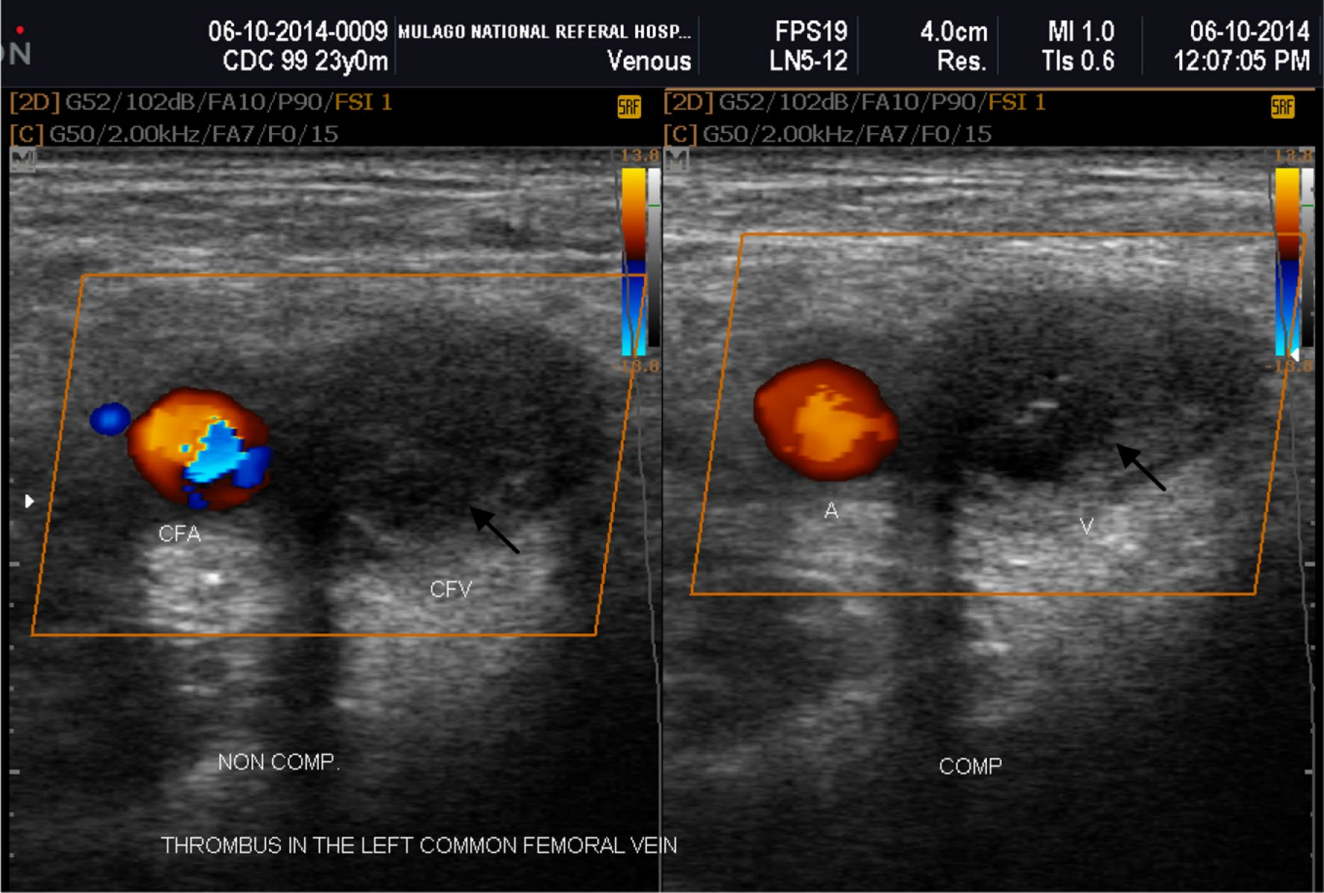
Acute DVT. Transverse sonogram of a 23 years old man on second line HAART who presented with acute DVT of CFV. Ultrasound scan shows lack of compressibility of the left CFV, presence of heterogeneous thrombus (black arrow) in the lumen and absence of spontaneous Doppler signal
